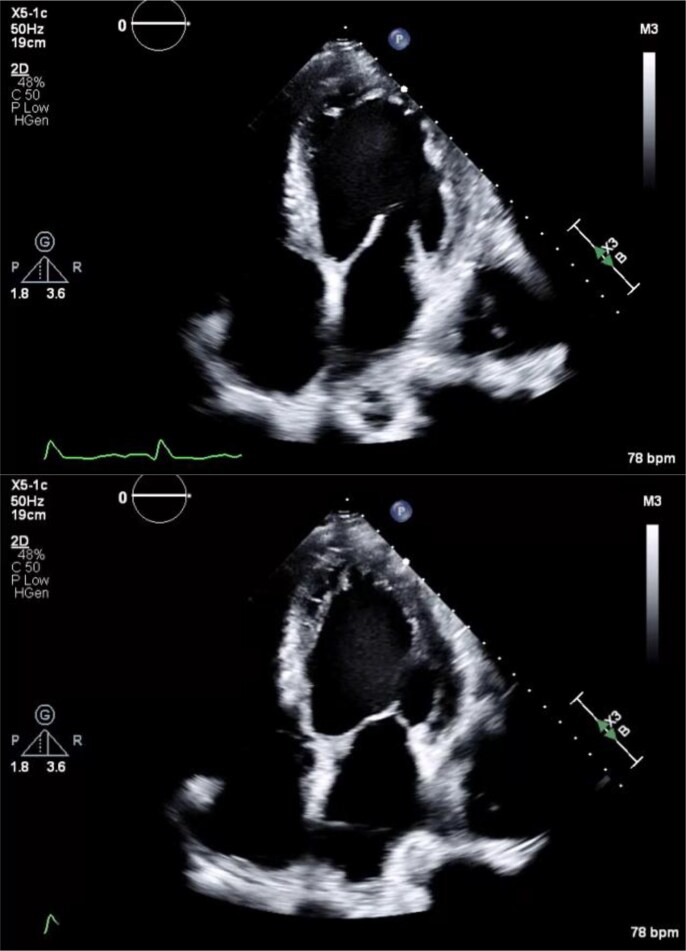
Transthoracic echocardiogram demonstrating severely dilated and dyskinetic left ventricle during diastole (top) and systole (bottom) for comparison
